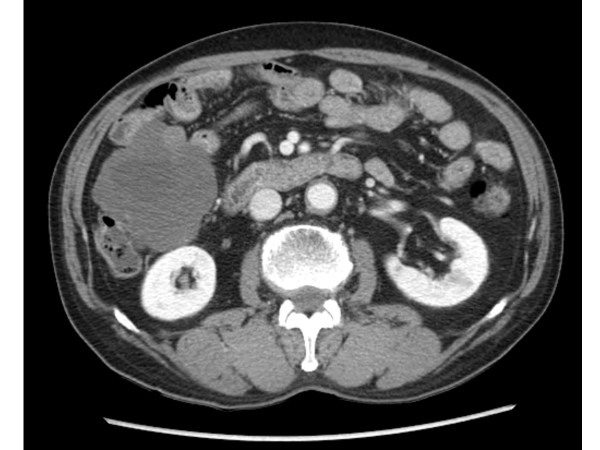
Last follow up CT scan revealed a partial response. The tumor decreased to 8 × 8 cm.
